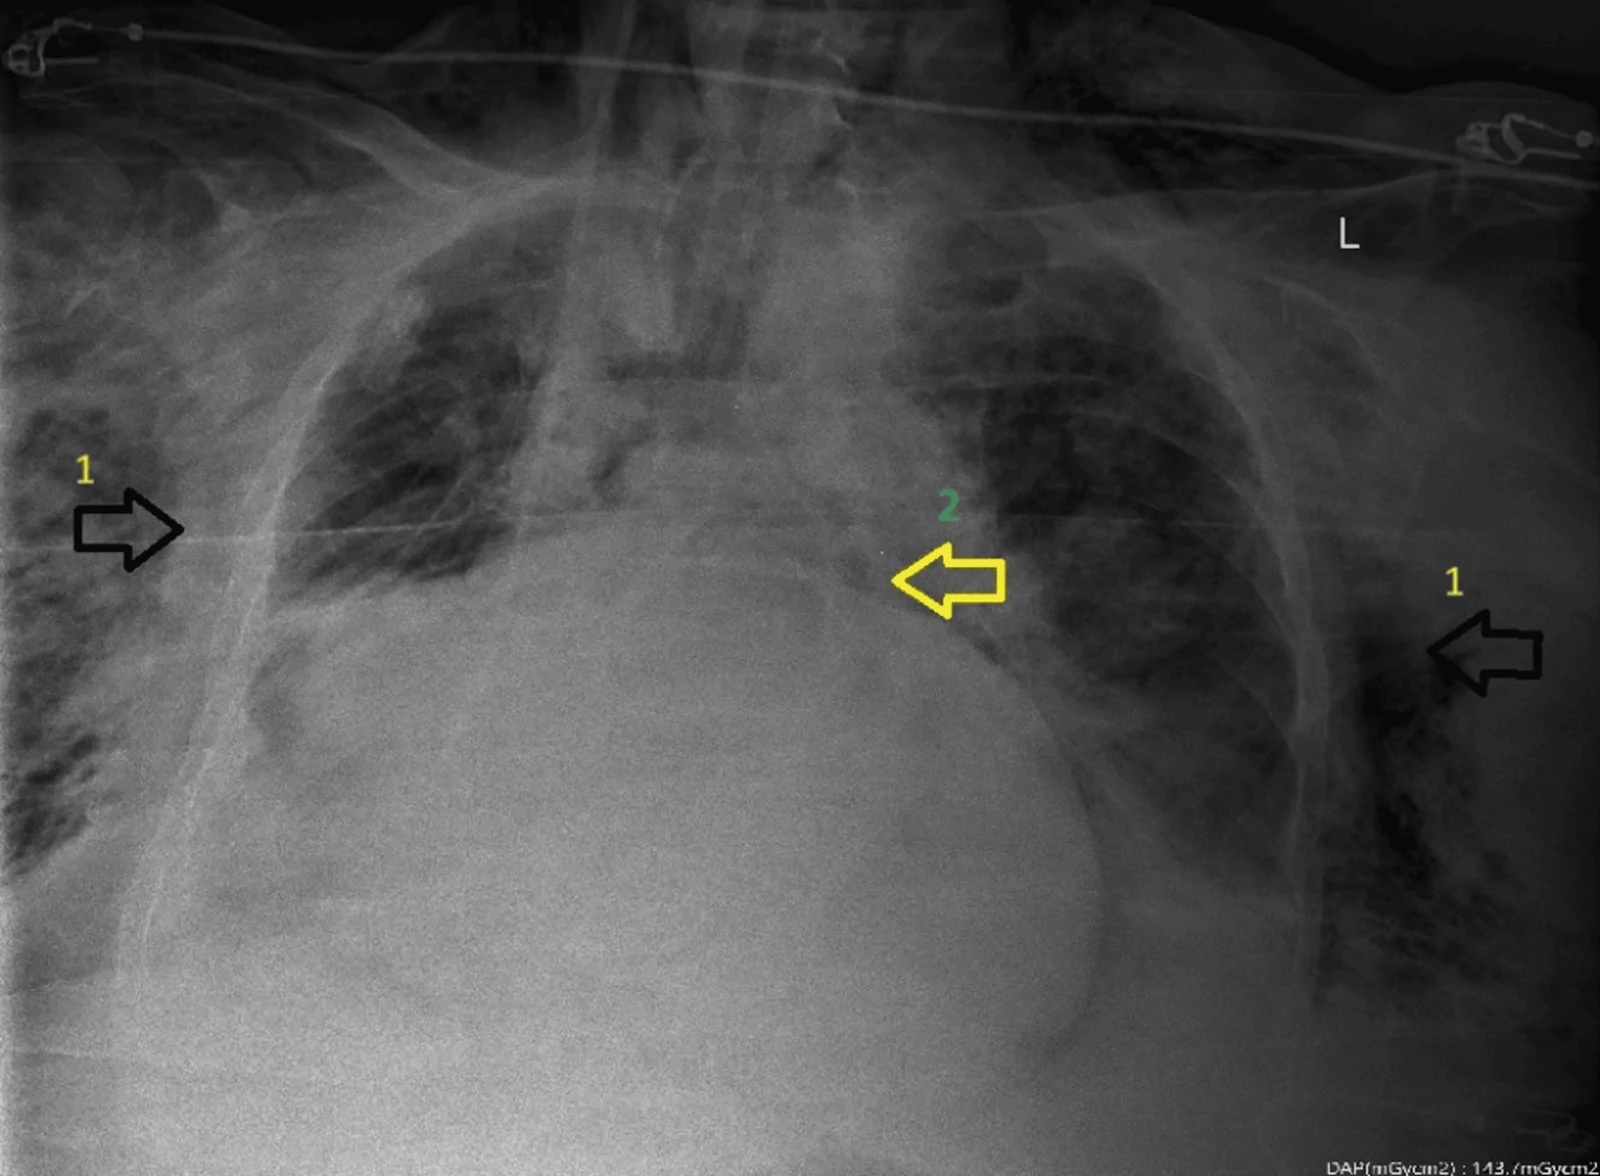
Supine anteroposterior chest radiograph Subcutaneous emphysema (arrow 1, bilateral) and pneumomediastinum (arrow 2).
